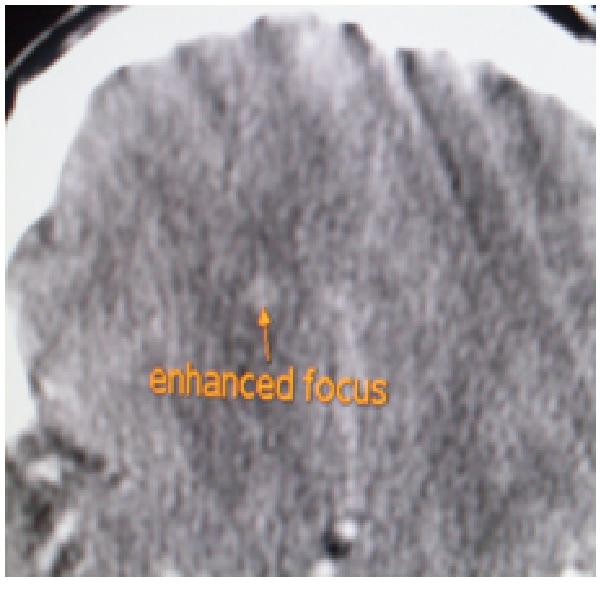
Axial computed tomographic image showing an enhancing intracranial focus.
